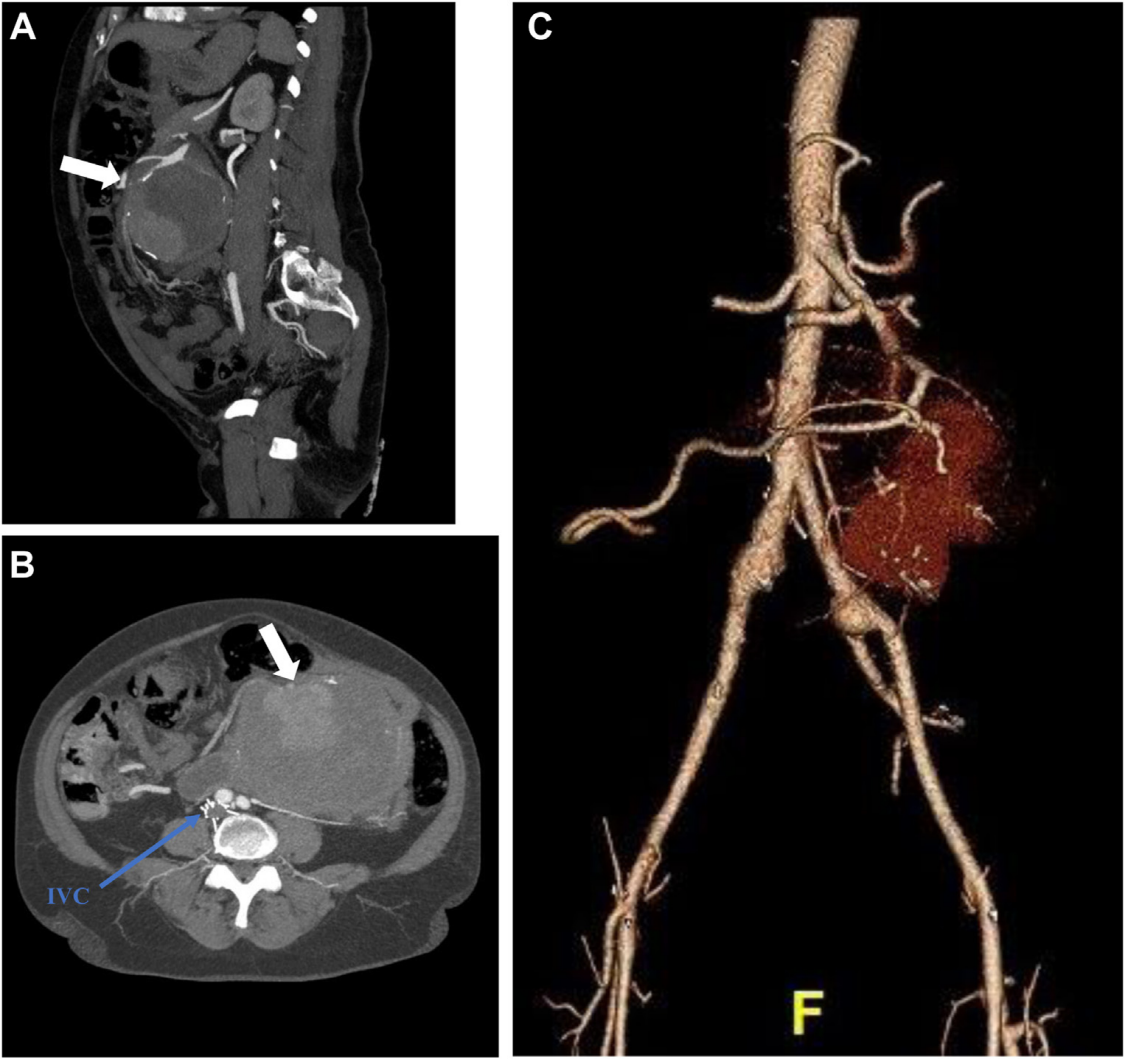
A-C, Sagital and axial view of abdominal computed tomography angiogram (CTA) demonstrating extensive surrounding mural thrombosis of a superior mesenteric artery (SMA) branch and feeding vessels (*arrow*). Three-dimensional preoperative reconstruction of SMA aneurysm. *IVC*, Inferior vena cava.
